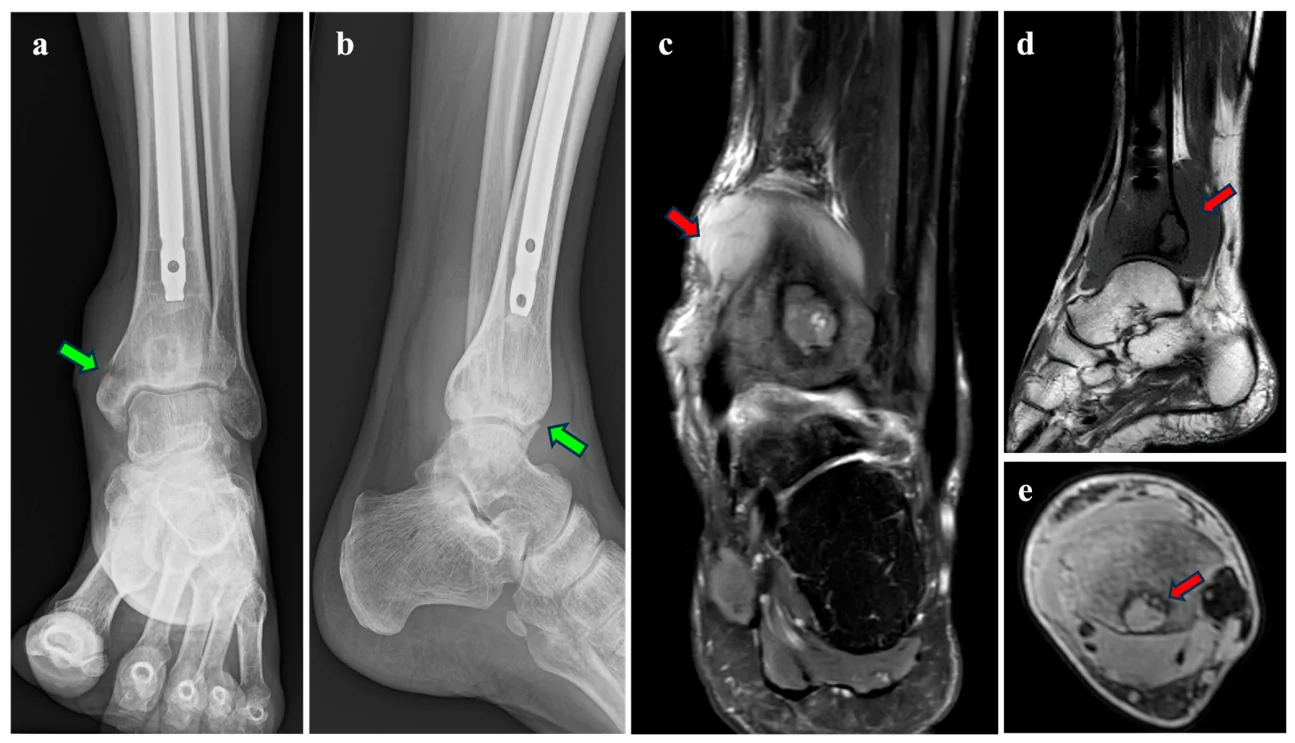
Anteroposterior and lateral radiographs obtained in July 2024 demonstrating a well-circumscribed lytic lesion associated with a pathological fracture of the medial malleolus (green arrow), as well as the intramedullary nail following screw removal for dynamisation (a,b). Contrast-enhanced MRI revealed enlargement of the distal tibial lesion compared with previous imaging, with a prominent soft-tissue component visible on all sequences (c–e) showing post-contrast enhancement (c), low signal intensity on T1-weighted images (d), and marked perilesional bone marrow oedema on T2-weighted sequences (e) (red arrows).
